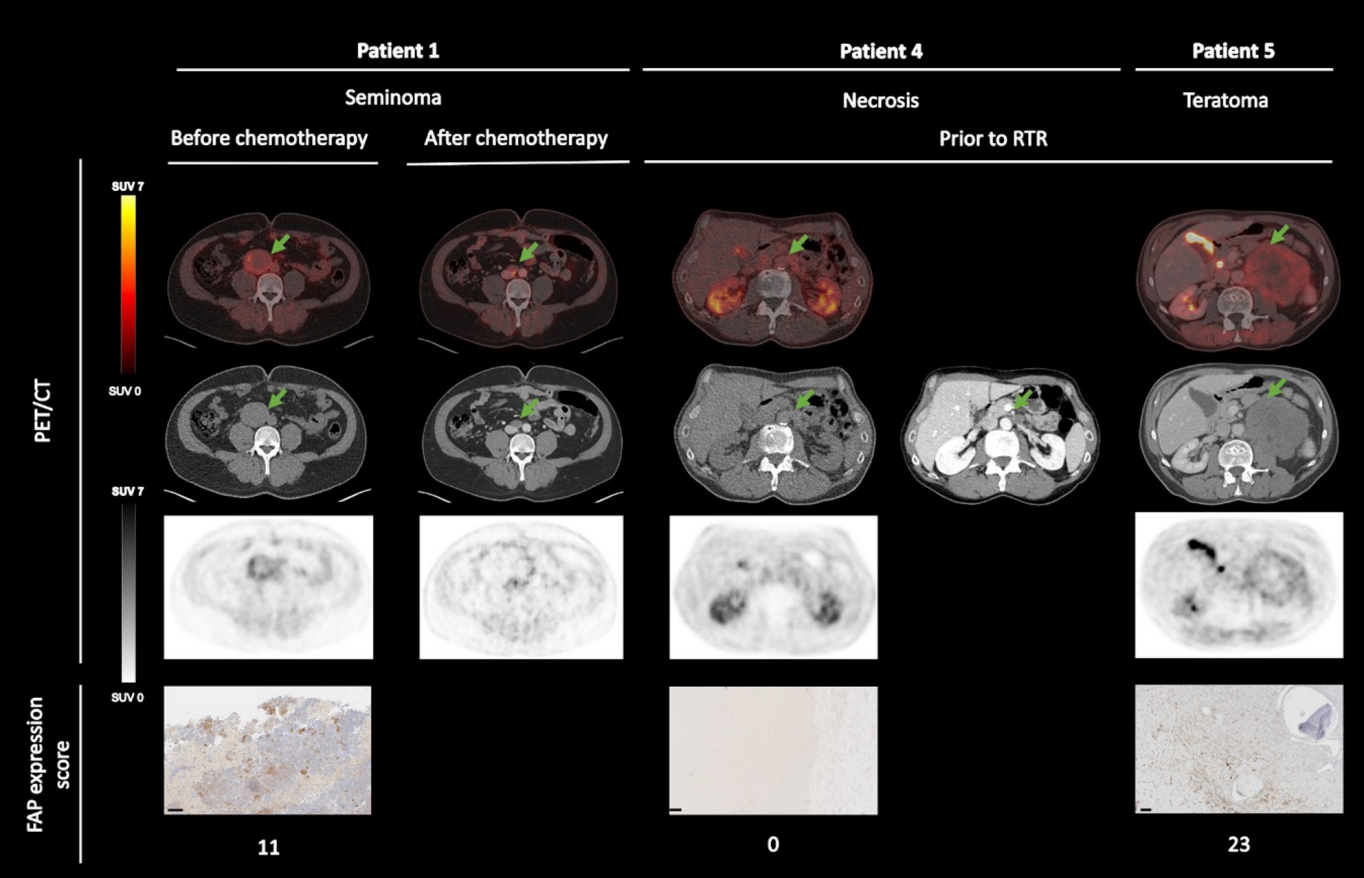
Results from PET/CT scan correlated with FAP expression score in pathology (RTR = residual tumor resection)
